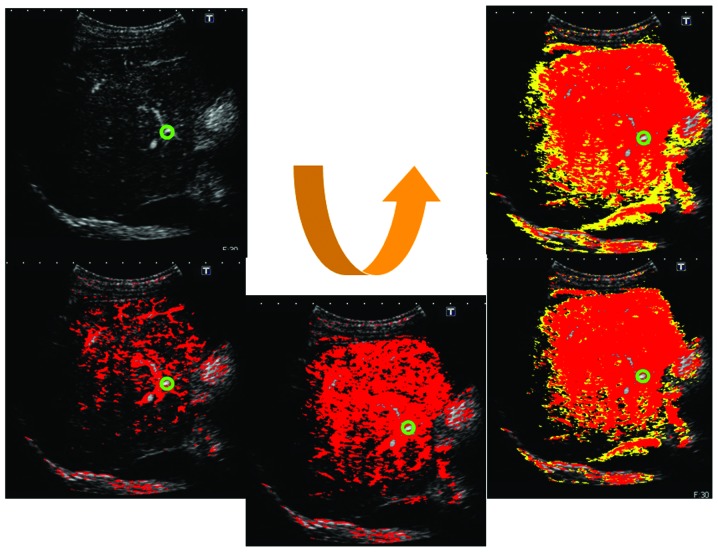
Creating arrival time parametric imaging (At-PI) images. With the large hepatic artery branch in the right hepatic hilum as the region of interest (ROI; the green circle), the ultrasonography system set the point at which the ROI was contrasted as time 0, sequentially calculated the arrival time of individual pixels in the hepatic parenchyma and automatically color coded B mode images. Red pixels indicate an arrival time of 0–5 sec, and yellow pixels indicate an arrival time of 6–10 sec.
